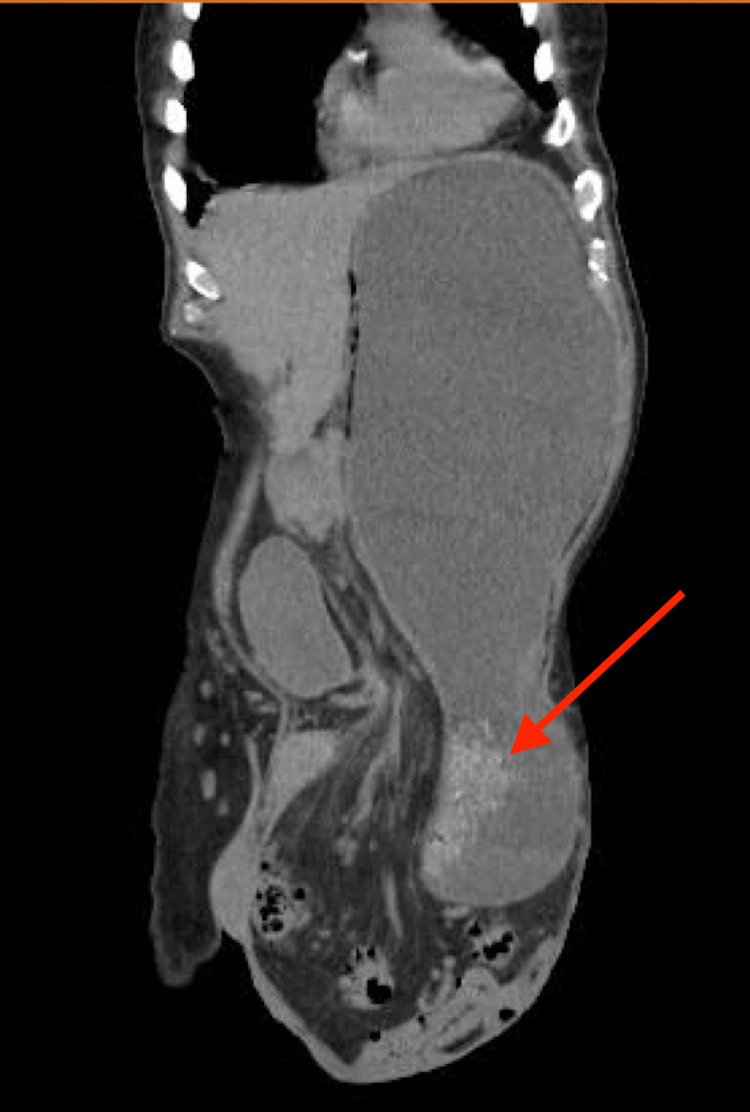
CT Abdomen and Pelvis Demonstrating Stomach in Hernia
